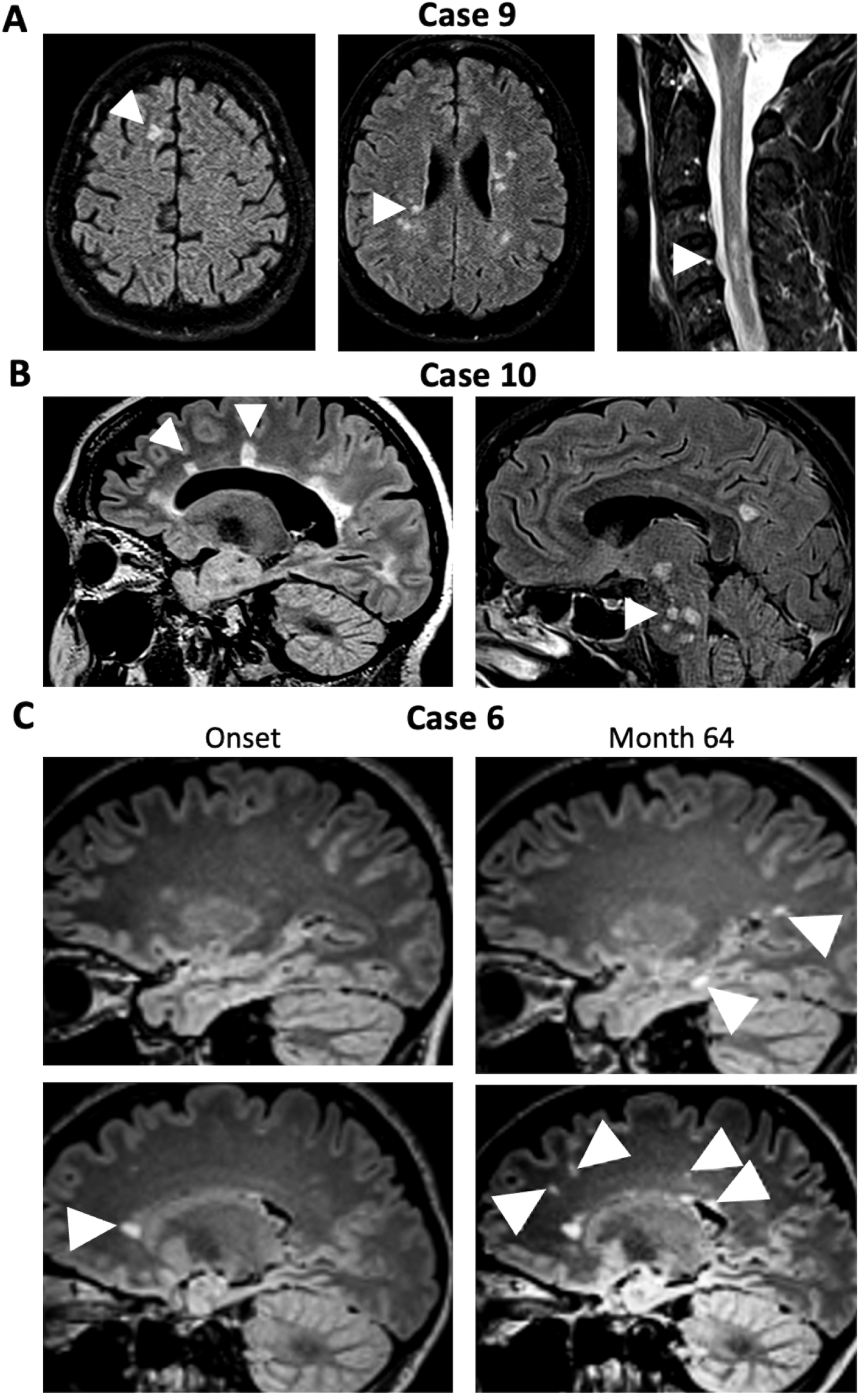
MRI in patients NMDAR-E with ataxia and concomitant or later diagnosis of multiple sclerosis. (A) Initial FLAIR cerebral MRI of Case 9 show juxtacortical (left panel, arrowhead) as well as periventricular lesions (middle panel, arrowhead) suggestive of demyelination. T2-weighted MRI of the cervical spinal cord also shows multiple demyelinating lesion (arrowhead). (B) Cerebral FLAIR MRI of Case 10 shows extensive periventricular T2-hyperintense lesions with Dawson finger-like orientation, highly suggestive of MS-like demyelination (left panel, arrowhead) as well as infratentorial lesions (right, arrowhead). (C) Initial cerebral FLAIR MRI of Case 6 (left panels) shows small unspecific subcortical white matter lesions but one periventricular lesion (arrowhead). Upon follow-up (Month 64, right panels) a juxtacortical T2-hyperintense lesion (upper right panel, lower arrowhead) as well as a new periventricular lesion (lower right panel, right arrowhead) in addition to multiple subcortical lesions (other arrowhead), some with radial orientation (lower right panel, left two arrowheads).
